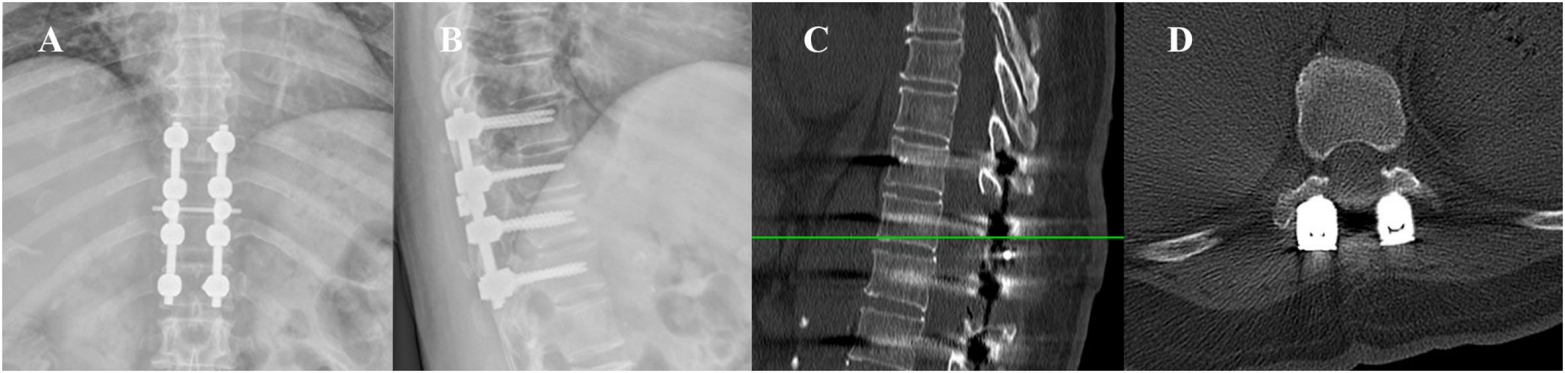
The postoperative examination of the patient. (A, B) DR revealed appropriate internal fixation; (C, D) CT images demonstrated no residual mass compressing the spinal cord.
